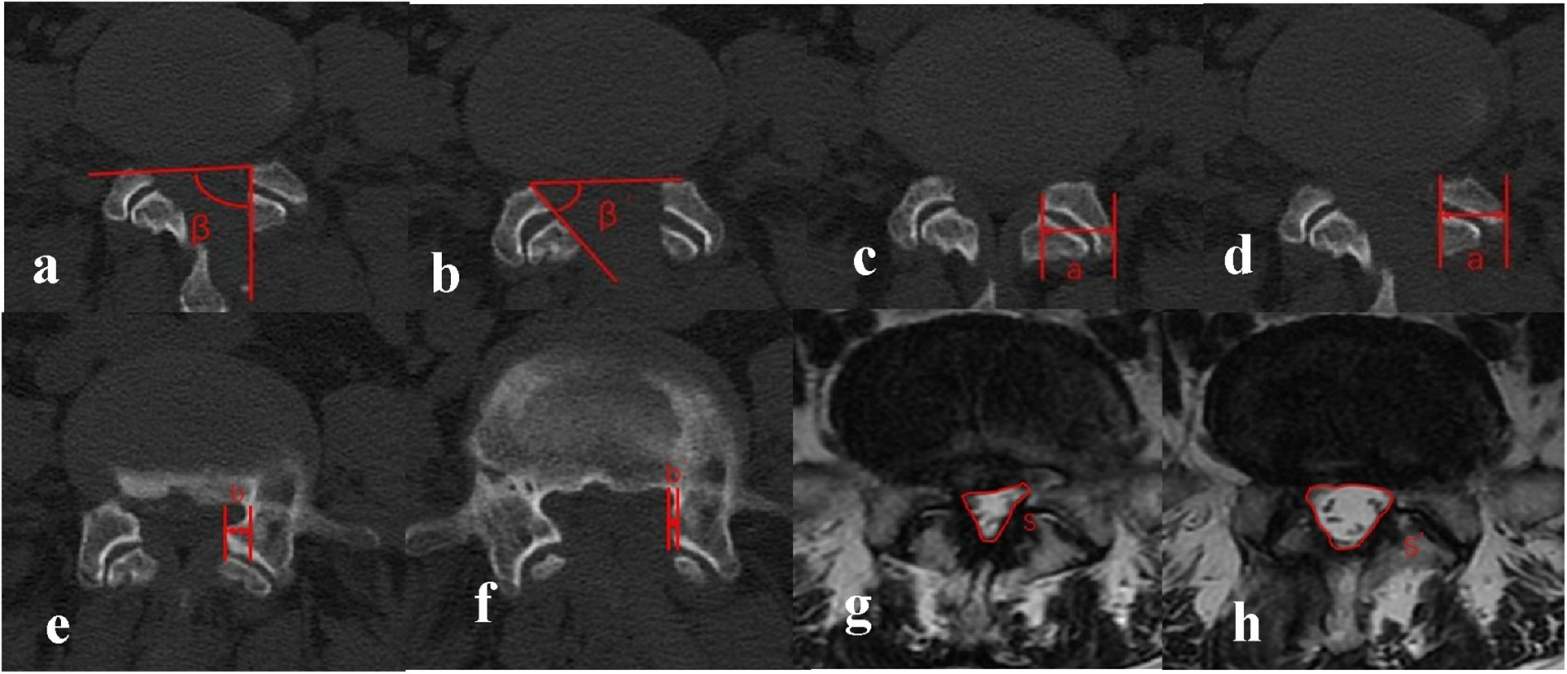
(a,b) medial facetectomy surface angle (MFSA): included angle between the line connecting the ventral vertices of the facet joints (FJ) on the two sides and the surgical tangent line of FJ, which was defined as the angle of MFS. β indicated angle at the ipsilateral side and β’ indicated angle at the contralateral side. (c,d) Facet Joint Preservation Rate (FJPR): The preoperative FJ width was denoted by a, and the postoperative FJ width was denoted by a’. The preservation rate of FJ = a’/a × 100%. (e,f) Lateral Recess Decompression Rate (LRDR): The preoperative distance from the medial border of the superior FJ to the medial border of the pedicle of vertebral arch was denoted by b, and the postoperative distance was denoted by b’. The decompression rate of LR = b’/b × 100%. (g,h) Dural Sac Cross-Sectional Area Expansion Rate (DSCAER): DSCAmeasured on the lumbar spine MRI was denoted by S, and the postoperative area was denoted by S’. The expansion rate of DSCA = S’/S × 100%.
